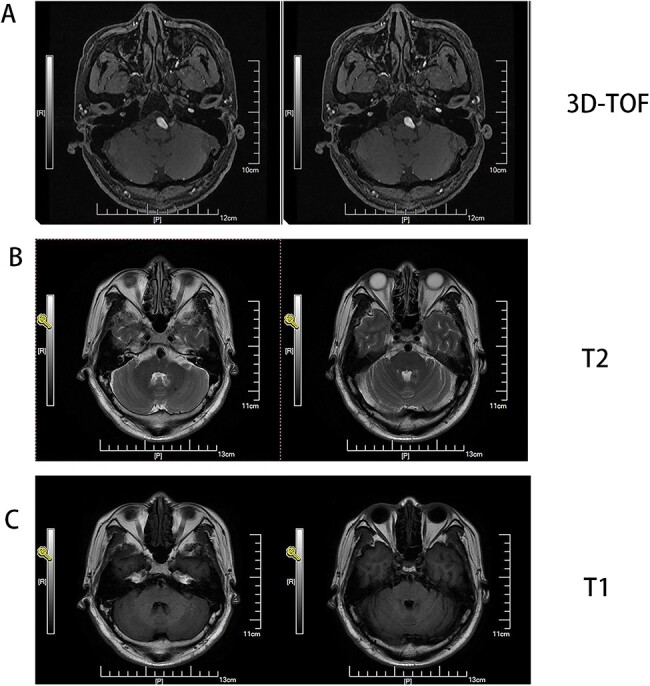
(A) MRI 3D-TOF(3 digital- time of flight), (B) MRI T2, C:MRI T1. Basilar trunk dilation up to 9 mm of diameter deviated path to the left side responsible of medulla compression.
